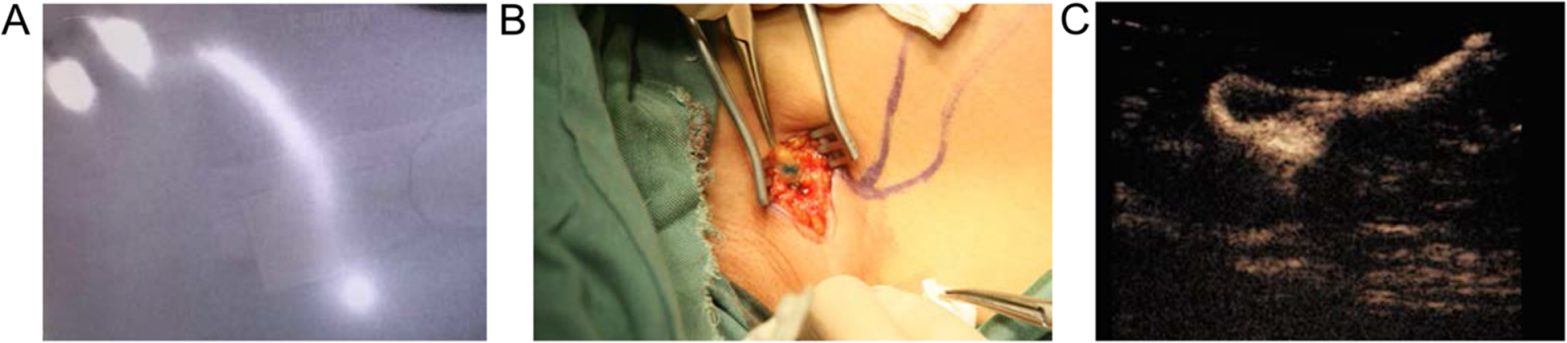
Recurrence-free survival in the contrast enhanced ultrasound (CEUS) + blue dye and indocyanine green (ICG) + blue dye groups
